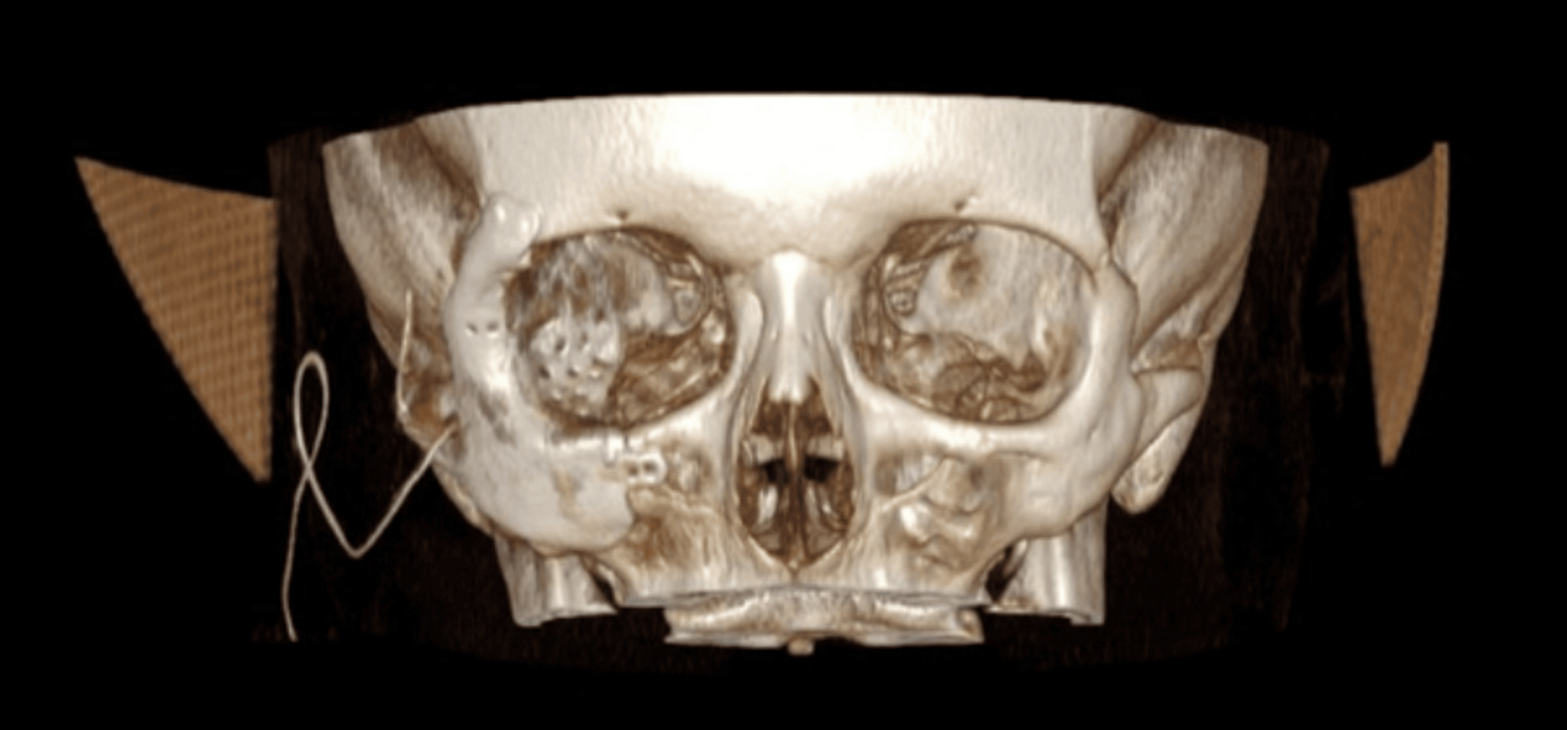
3D frontal view of CT head with right orbito-zygomatic implant
